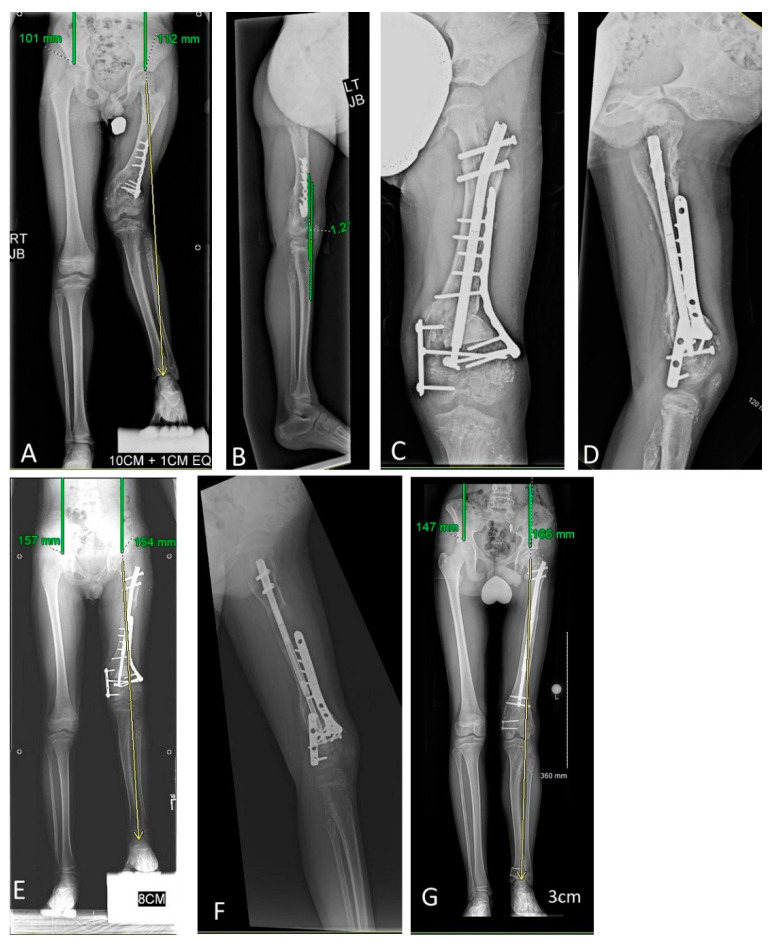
Acute coronal plane deformity correction with simultaneous intramedullary motorized nail lengthening. This figure portrays Patient 7’s first lengthening experience. (A) An 8-year old male with enchondromatosis predominantly affecting the left femur, radiographically consistent with Ollier’s disease. Left leg shorter than right by 122 mm, predicted to be 184 mm at maturity. Coronal full leg length radiography identifies additional substantial left genu valgus deformity mainly occurring in the distal femur. (B) Lateral radiograph identifies no sagittal plane deformity requiring correction. (C,D) Anterior–posterior and lateral immediate postoperative radiographs demonstrating acute coronal correction via an osteotomy and plate internal fixation strategy, with simultaneous lengthening nail inserted with a proximal osteotomy where lengthening will occur. (E) Full length standing radiograph taken upon completion of 50 mm uncomplicated lengthening. The coronal deformity is improved. (F) The patient is suboptimally positioned for the lateral radiograph which was not repeated at the time, but is shown to demonstrate no sagittal plane deformity occurred through the femur during the lengthening process. (G) The patient’s current full length radiograph after his third lengthening identifies 41 mm residual LLD, with slight genu valgum progression compared to the initially provided correction. A final lengthening surgery with repeat acute coronal plane correction is planned following skeletal maturity.
